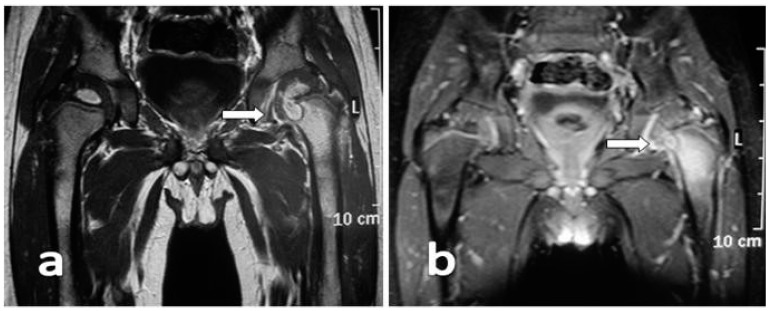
MRI confirms osteomyelitis of the femoral neck with a possible involvement of the growth plate without involvement of the joint cavity (arrow). (a) T1-weighted image showing metaphyseal fluid collection with surrounding edema; (b) T1-weighted SPIR image enhancing the fluid component.
